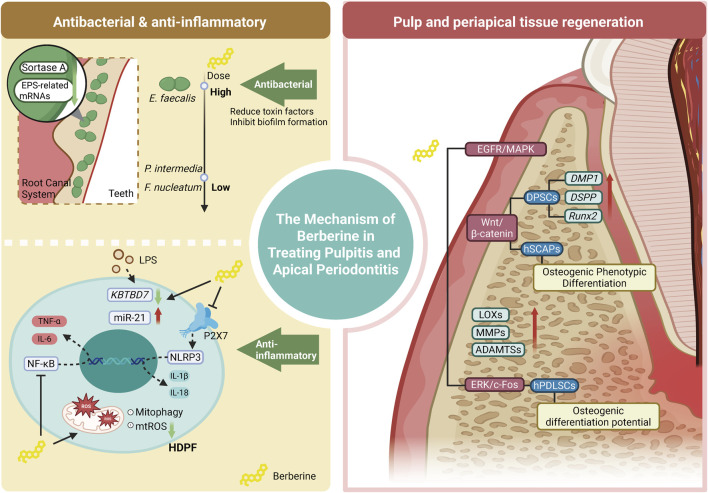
Molecular Mechanisms of Berberine in Pulpitis and Apical Periodontitis. Antibacterial and anti-inflammatory effects (Left) and Pulp and periapical tissue regeneration (Right). *Enterococcus faecalis* (*E. faecalis*), *Prevotella intermedia (P. intermedia)*, *Fusobacterium nucleatum (F. nucleatum),* Mitochondrial reactive oxygen species (mtROS), Human dental pulp fibroblasts (HDPFs), Human periodontal ligament stem cells (hPDLSCs), Lysyl oxidases (LOXs), Matrix metalloproteinases (MMPs), A disintegrin and metalloproteinase with thrombospondin motifs (ADAMTS), and Dental pulp stem cells (DPSCs). Created in BioRender.
